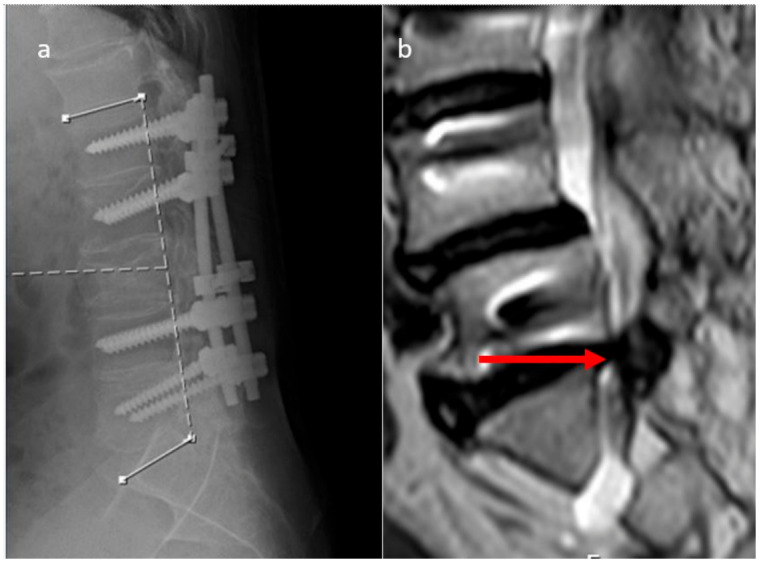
Distal adjacent segment disease associated with postoperative sagittal profile loss in a 60-year-old woman. (a) Lateral radiograph showing flattening of the lumbar sagittal profile after posterior instrumentation, consistent with a postoperative flatback-type alignment pattern. This configuration may increase compensatory mechanical demand at the remaining caudal mobile segment. (b) Sagittal magnetic resonance image demonstrating distal adjacent-level disc disease and stenosis below the instrumented construct. This case highlights that ASD surveillance after thoracolumbar/lumbar fusion should not be limited to the proximal junction; distal mobile segments may also become mechanically vulnerable, particularly when lumbar lordosis is reduced, and caudal motion segments are required to compensate. The dotted line indicates the reference alignment line used for radiographic assessment, the white line indicates loss of lumbar lordosis as generated by the PACS measurement tool, and the red arrow indicates the relevant distal adjacent-segment pathology.
